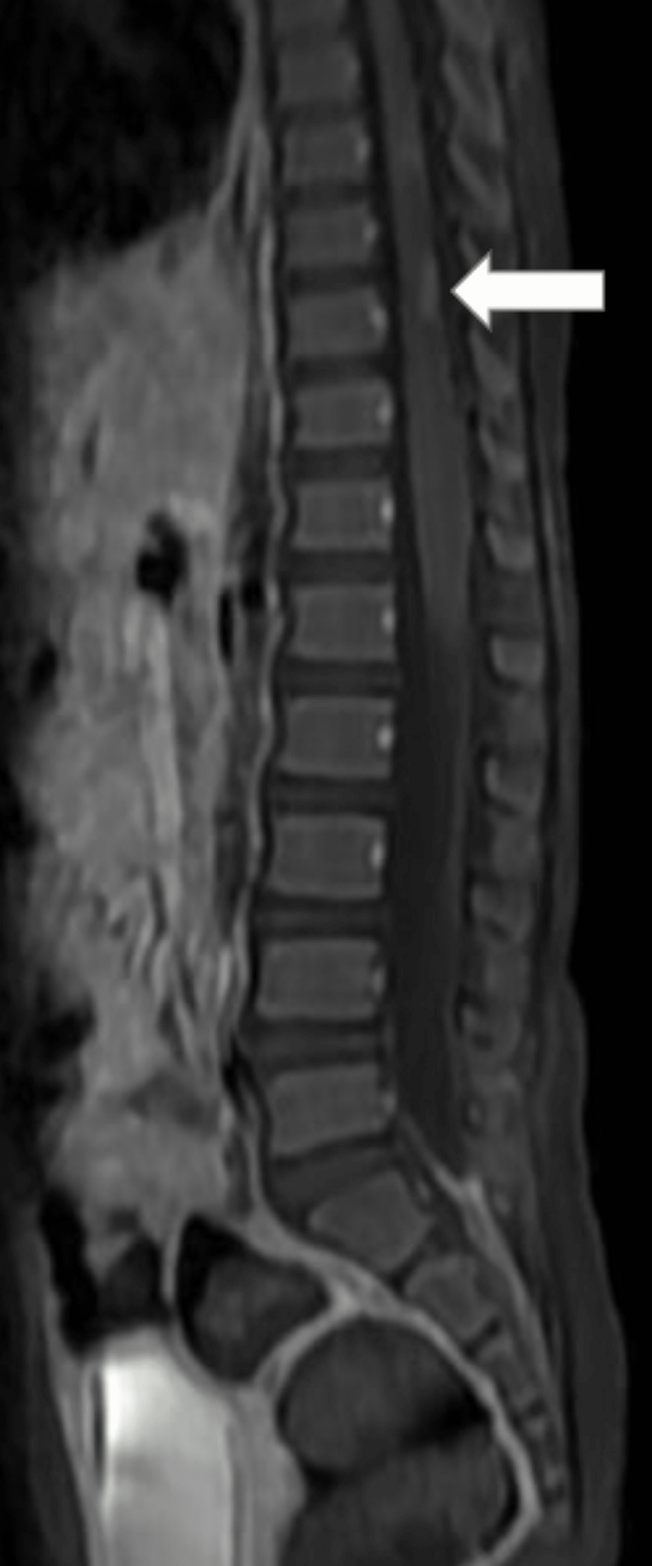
Saggital MRI showing lesion at the D10 level. MRI: magnetic resonance imaging; D10: dorsal vertebrae
